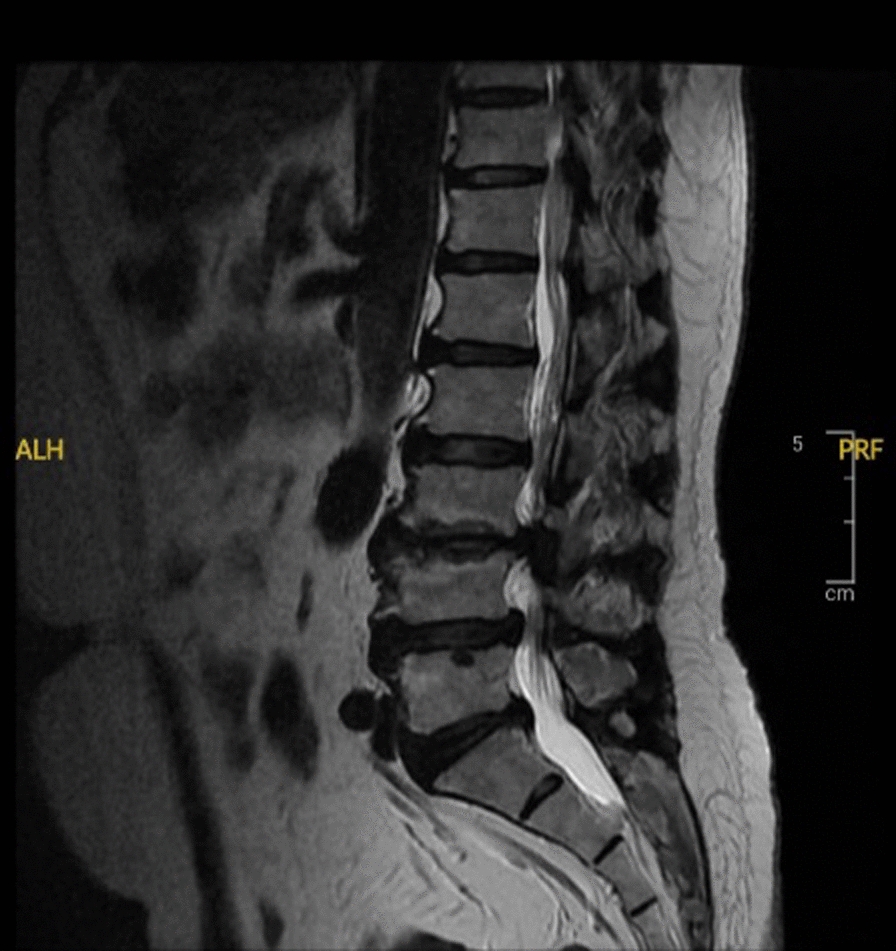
Lumbosacral magnetic resonance imaging of patient I
